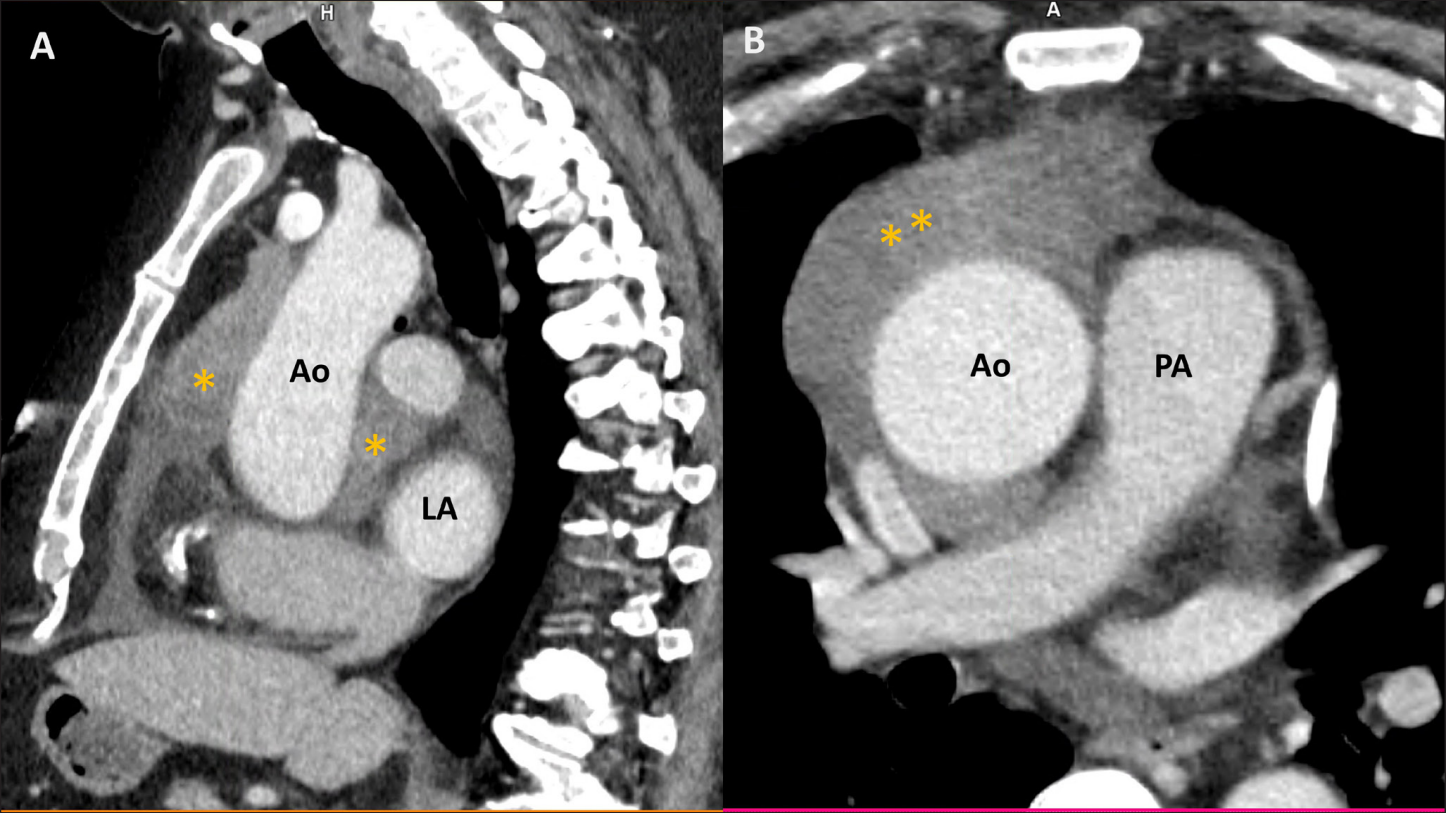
Contrast-enhanced computed tomography scan of the chest, sagittal view (A), and axial view (B) showing a hyperintense (Housefield unit of 50) large lobulated anterior mediastinal mass (asterisks) encasing the aorta and extending posteriorly to the left atrium. Ao: aorta; LA: left atrium; PA: pulmonary artery
